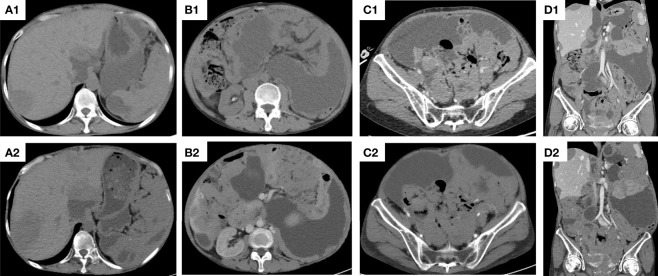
The computed tomography (CT) of the patient. (A1) Upper abdominal CT scan before apatinib treatment; (A2) Upper abdominal CT scan after apatinib treatment for 3 years; (B1) Lower abdominal CT scan before apatinib treatment; (B2) Lower abdominal (CT) scan after apatinib treatment for 3 years; (C1) Pelvic CT scan before apatinib treatment; (C2) Pelvic CT scan after apatinib treatment for 3 years; (D1) Coronal CT scan before apatinib treatment; (D2) Coronal CT scan after apatinib treatment for 3 years.
